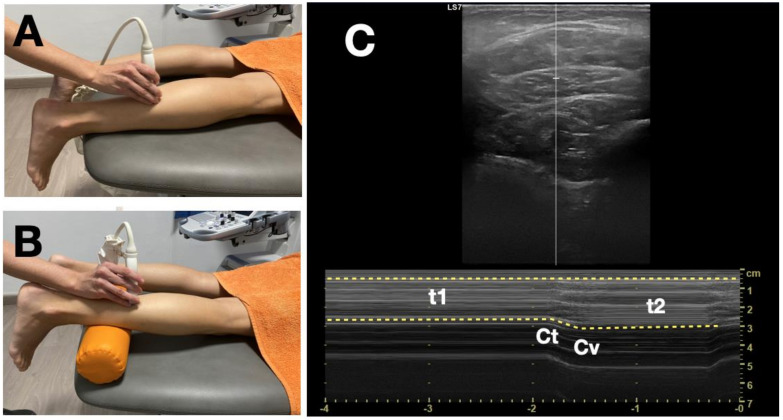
(A) Participants with their knees extended. (B) Participants with their knees flexed at 30°. (C) Ultrasound image of the soleus muscle displayed in M-mode during maximal isometric ankle plantar flexion measurements: t_1_, initial thickness; t_2_, end thickness; Ct, contraction time and Cv, contraction velocity.
